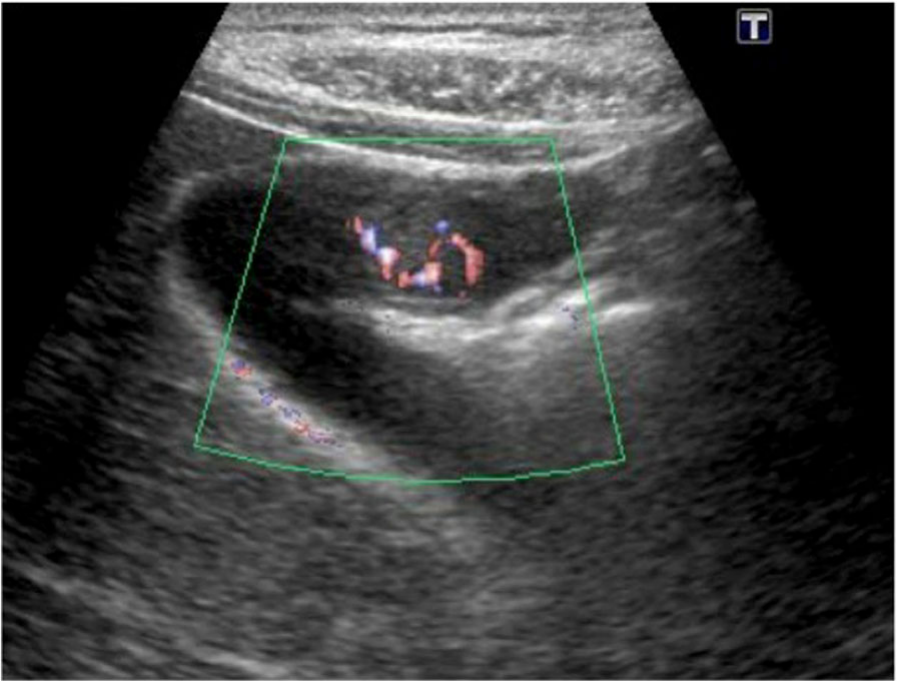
Color Doppler US images revealed hypervascular tumor of the gallbladder
